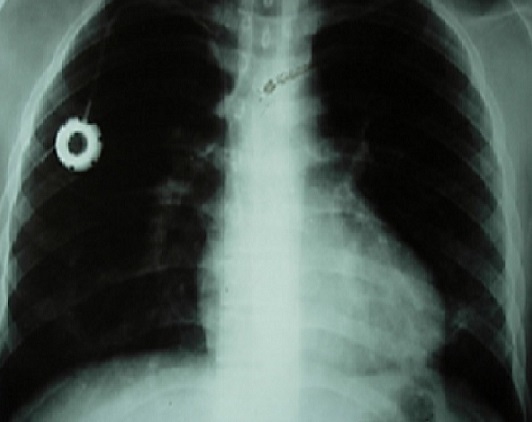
Radiological recovery of the patient on chest X-ray
